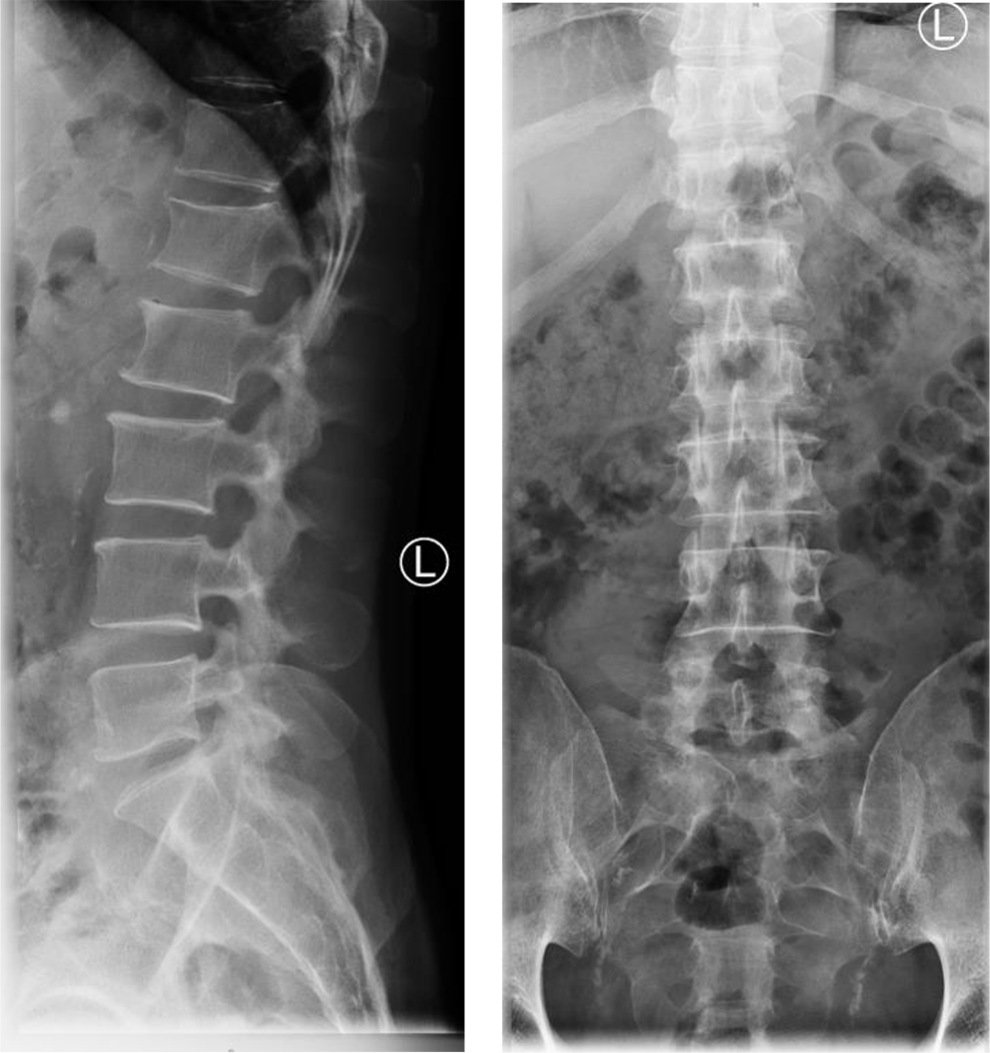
59 years, male, fall from motorcycle, TH12, L1 and L3 fractures AO type A1, clear indication for conservative treatment and moderate pain, no absolute indication for CT and MRI
